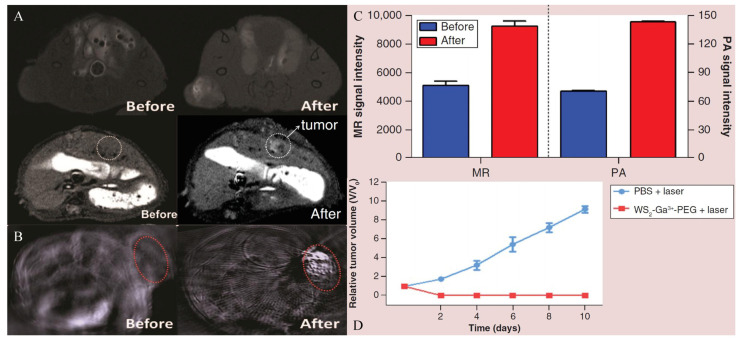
WS_2_-Ga^3+^-PEG-peptide nanoprobe served as a bimodal imaging and photothermal agent. Reproduced with permission Ref. [47]. Copyright 2018 Future Medicine LTD. (A) The MR imaging in subcutaneous and orthotopic HCC models. (B) The photoacoustic imaging in subcutaneous HCC models. (C) The quantified signal intensities of MR and photoacoustic imaging. (D) Effect of photothermal therapy in subcutaneous HCC models.
